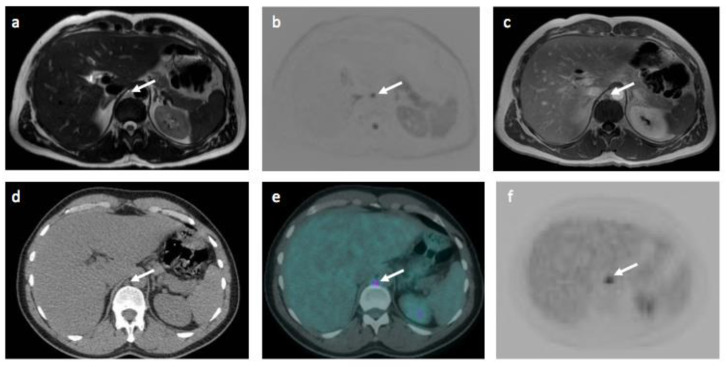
Images of 46-year-old male patient with Hodgkin’s lymphoma highlighting a false negative technical error on WB-MRI. A sub-centimeter FDG avid (SUV_max_ 5.1) retrocrural lymph node that was considered negative nodal site on WB-MRI. The positive nodal station is shown (arrows) on (a) T2-TSE, (b) DWI b1000 and (c) Contrast-enhanced in-phase mDixon WB-MRI images and (d) CT scan, (e) fused ^18^F-FDG PET-CT and (f) ^18^F-FDG PET images.
